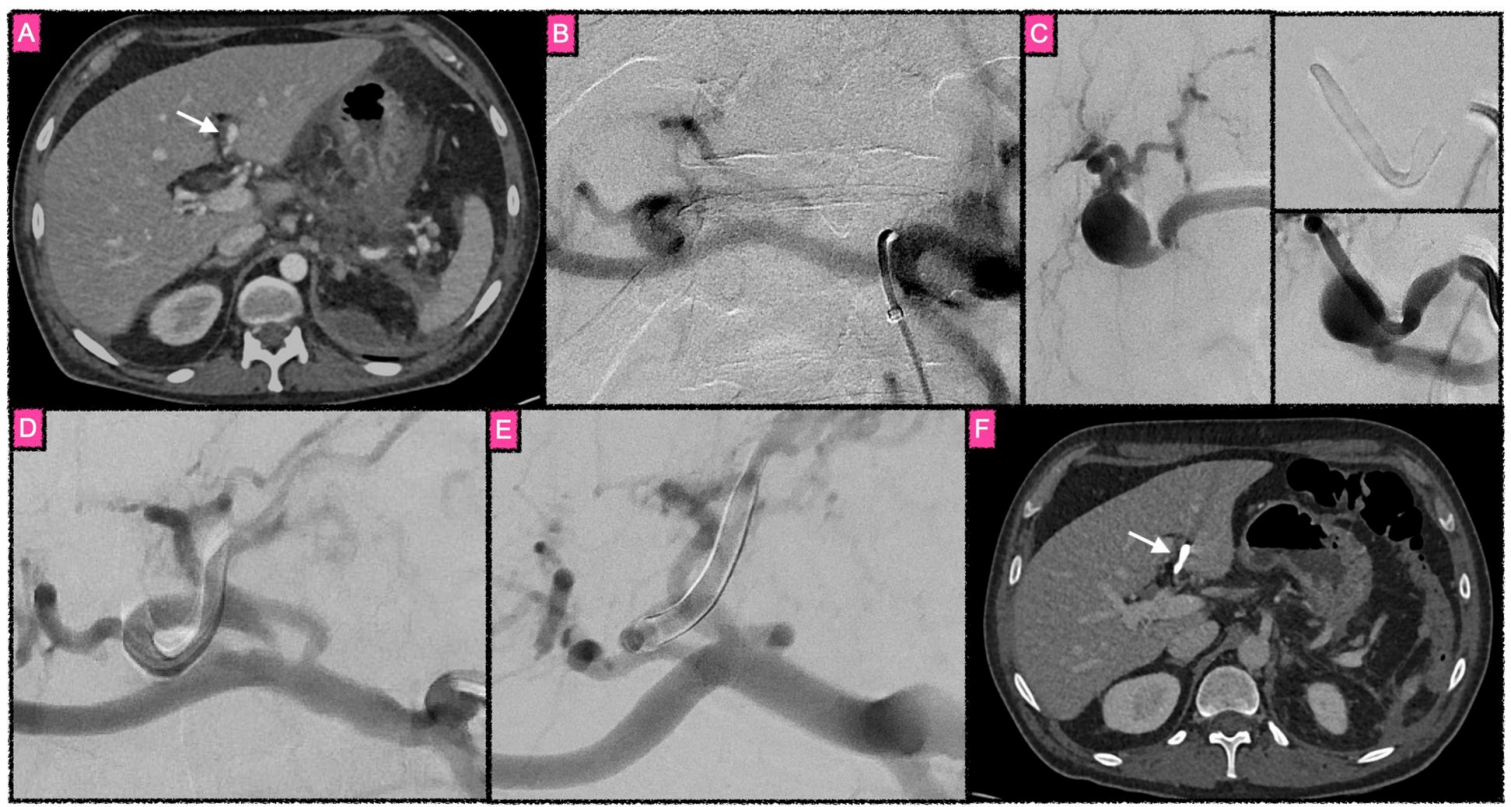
Treatment of an inflammatory aneurysm of the left hepatic artery. (A) Transversal computed tomography image in the early portal venous phase reveals a partially thrombosed aneurysm of the proximal left hepatic artery as a consequence of acute pancreatitis (white arrow). Follow-up ultrasound imaging (not shown) demonstrated rapid growth of the aneurysm within one week after the CT was performed, which was triggered by an episode of acute pain in the right upper quadrant of the patient's abdomen. Given the high risk for rupture and the anatomy at hand, endovascular treatment was selected. (B) Irregular aneurysm at the proximal left hepatic artery. (C) Course of FD treatment. The left side of (C) represents a microcatheter DSA run of the parent vessel prior to the implantation. There is significant vasospasm just proximal to the aneurysm, indicative of a previous rupture of the aneurysm. The right upper image in (C) shows the stent-in-stent construct within the parent vessel. A total of four FDS were implanted in an overlapping fashion in order to maximize the flow-diverting effect. (D) Early occlusion of the aneurysm after finalizing the multi-FDS implantation. The strong FD effect of the four implants in telescoping technique caused the almost immediate complete thrombosis of the aneurysm. (E) Follow-up DSA 3 months after treatment. The aneurysm is completely isolated from the circulation, the parent vessel remains patent. (F) Axial CT image 3 months after treatment, with the aneurysm thrombosed and shrunken (white arrow).
